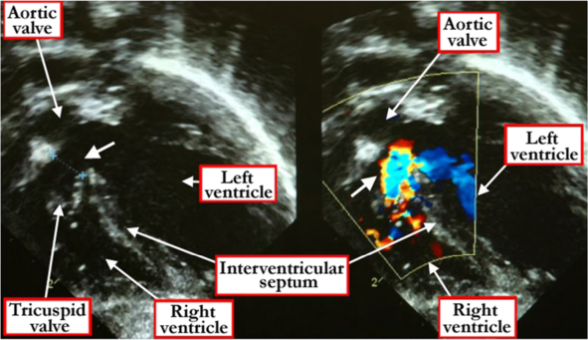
The subxyphoid four chamber cross-sectional echocardiogram shows the fibrous continuity between the leaflets of the aortic and tricuspid valves that reveals the defect to be perimembranous (white arrow) and opening to the inlet of the right ventricle (left hand panel). The right hand panel shows the color Doppler flow demonstrating the right-to-left shunt.
